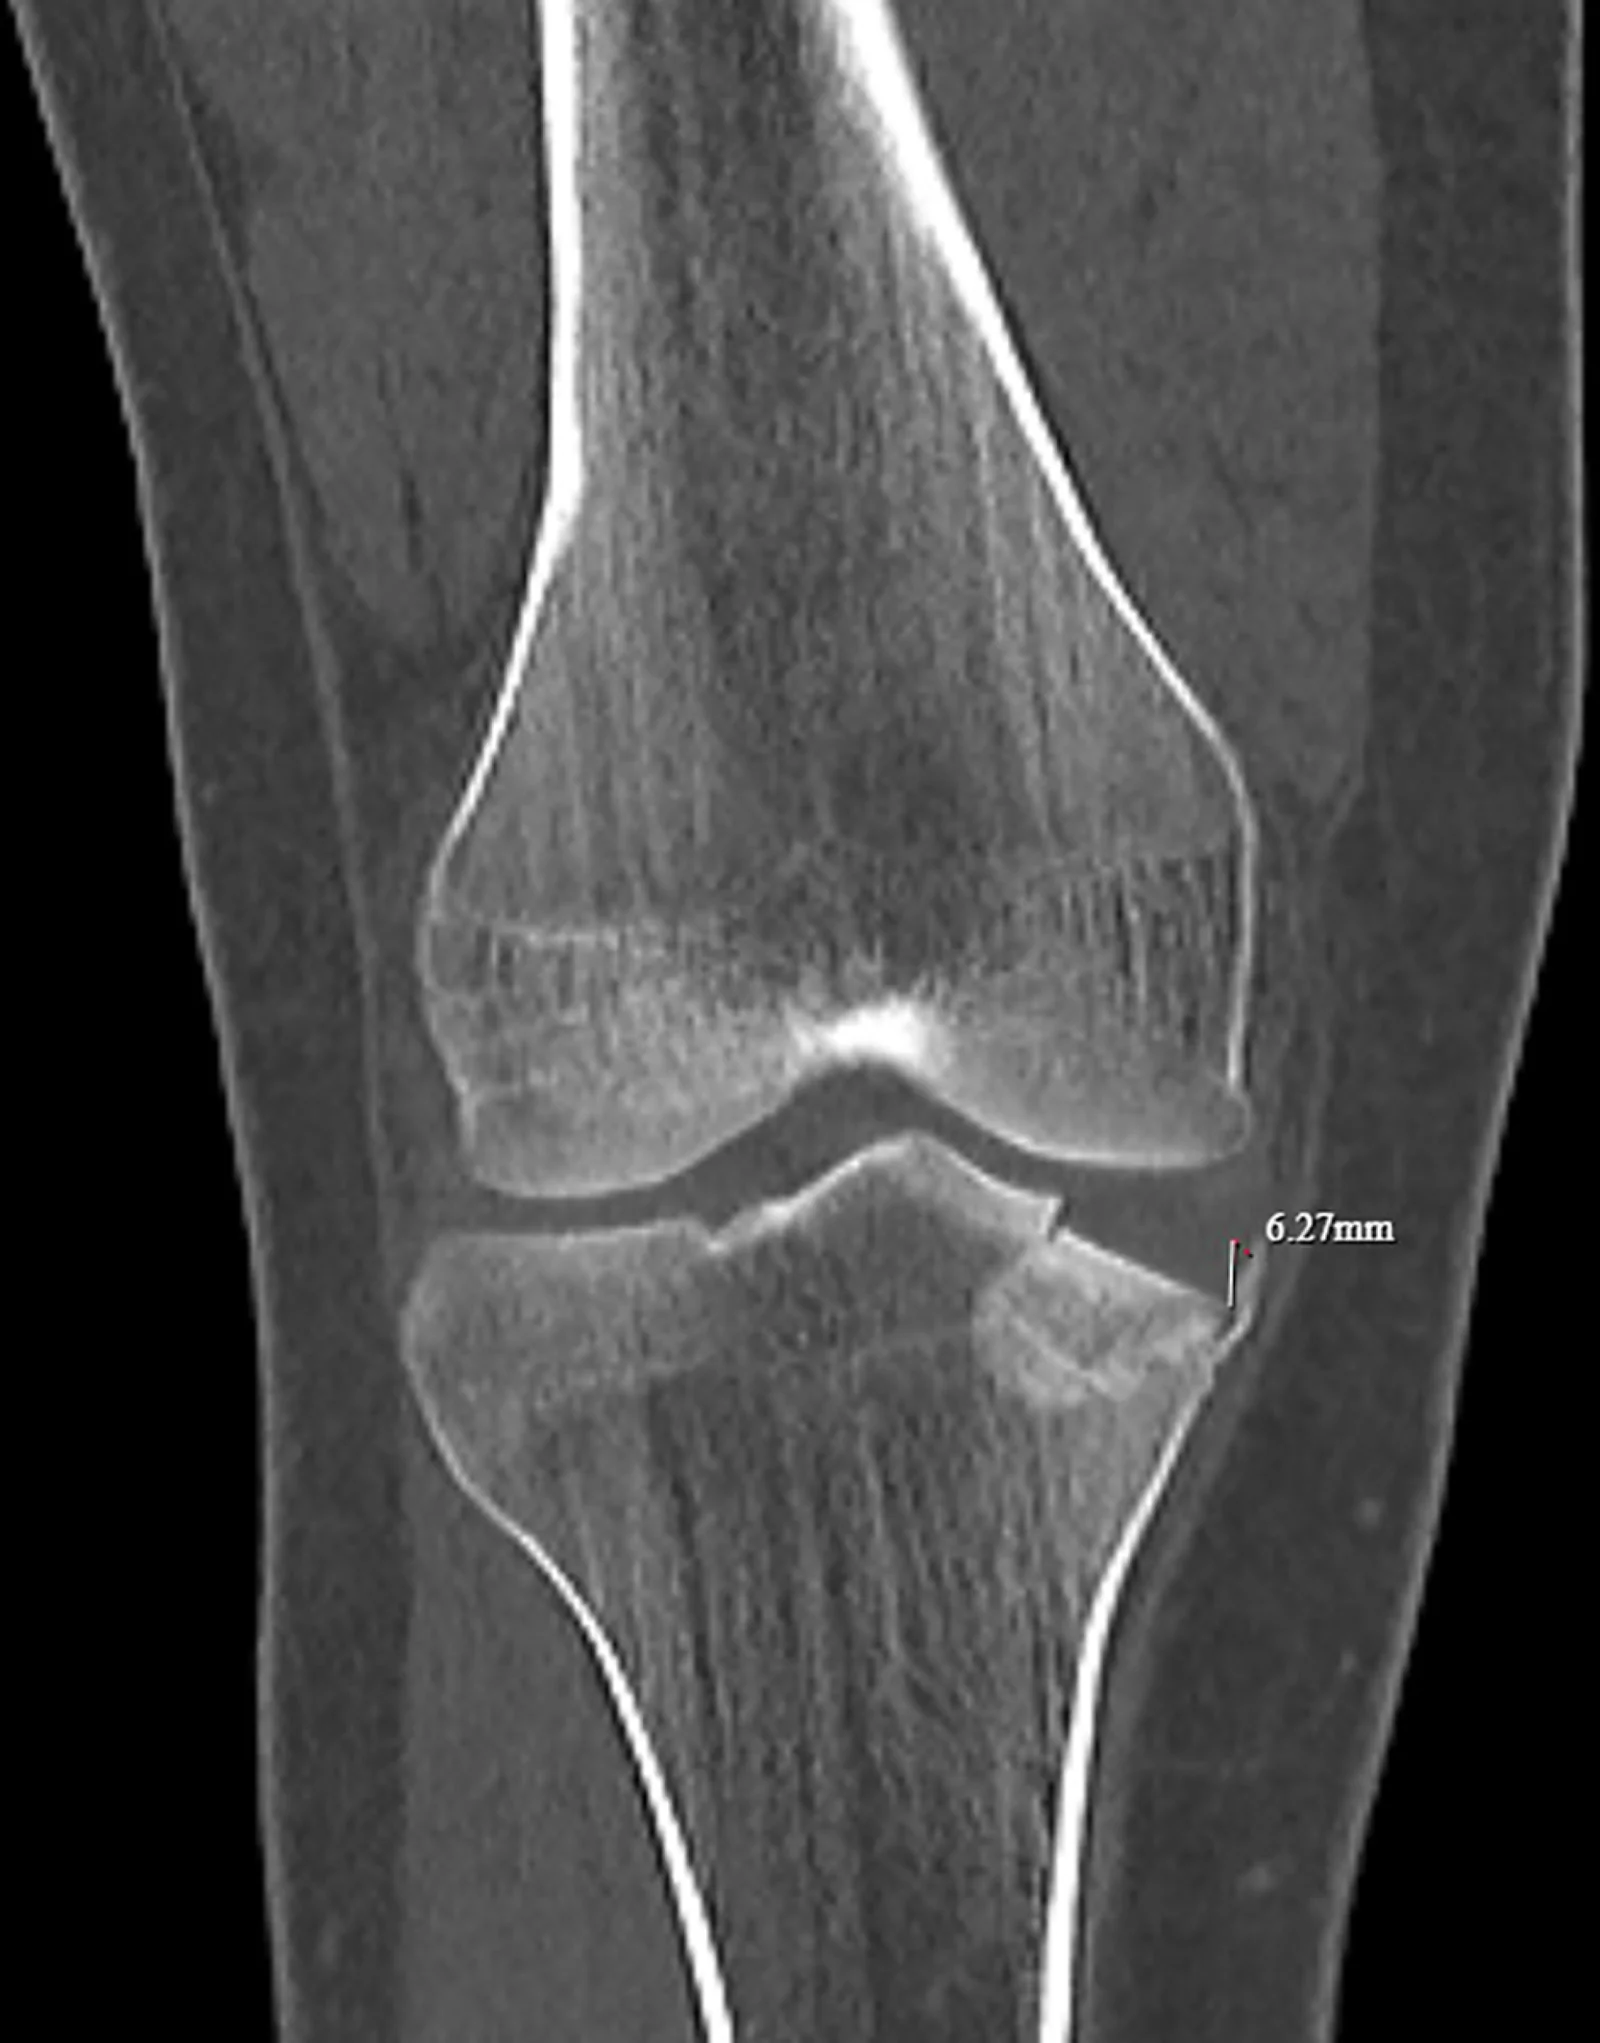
Preoperative CT. Coronal CT image confirming an isolated medial tibial plateau depression fracture with minimal displacement (≤ 10 mm), allowing accurate assessment of the articular depression and preoperative planning. CT = computed tomography.
